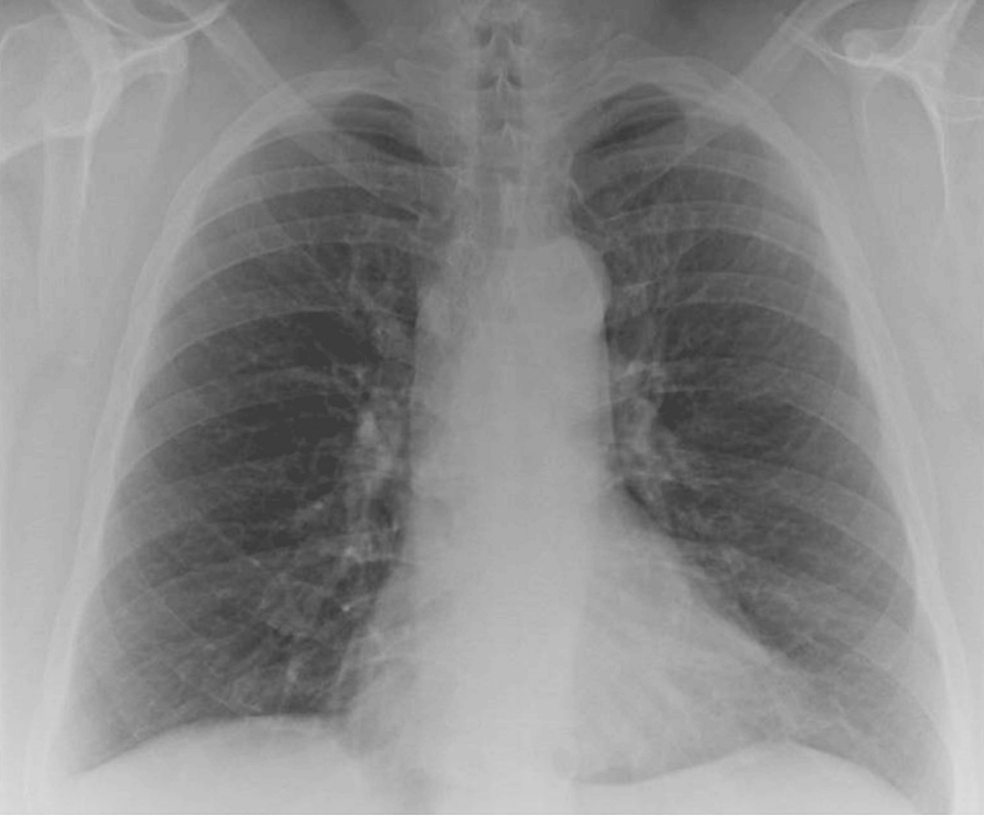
Post-treatment chest radiograph showing significant improvement of bilateral diffuse interstitial opacities.
